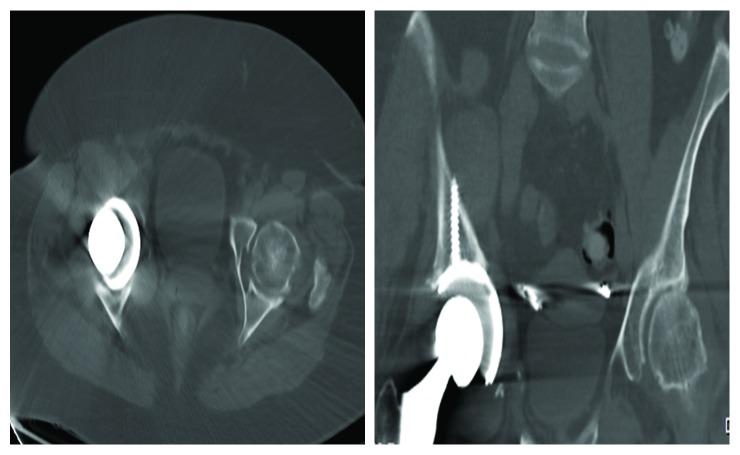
Axial and coronal CT scan showing excessive medialization of the acetabular component.
